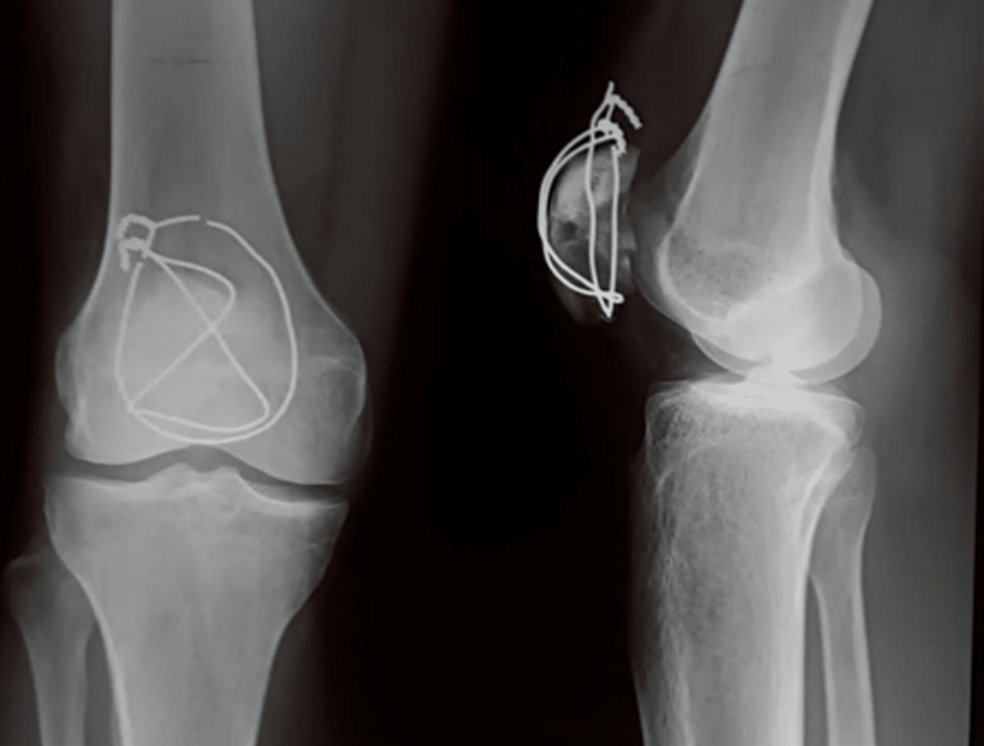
X-ray showing broken cerclage wire at 18 months of follow-up in one case
